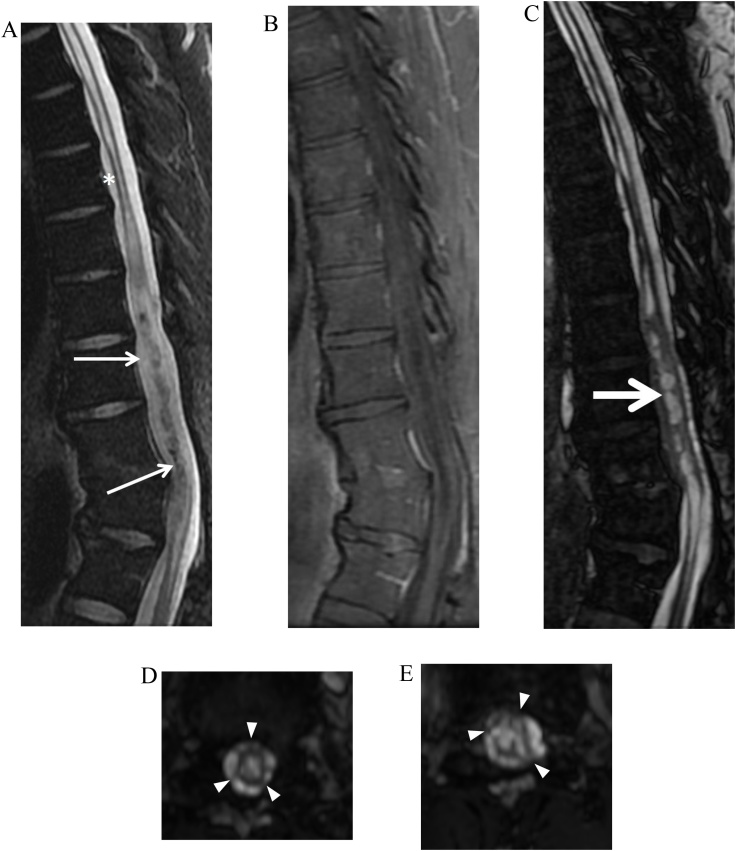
Arachnoid adhesions and complex syringohydromyelia. 34-year-old man with history of remote discitis osteomyelitis presents with worsening back pain, bilateral lower extremity weakness, and imbalance. Sagittal T2-weighted FSE MRI image (slice thickness, 3 mm) (a) demonstrates syringomyelia throughout the thoracic cord with myelomalacia cranially (asterisk) and relative expansion of the lower thoracic cord with central low signal (small arrows) which could be interpreted as a mass. Sagittal contrast enhanced T1-weighted FSE image (slice thickness, 3 mm) (b) demonstrates no abnormal enhancement. Sagittal (slice thickness, 1.2 mm) and axial FIESTA-C MRI images (c–e) clearly demonstrate the complex syrinx in the expanded lower cord with multiple internal septations (large arrow). Multifocal regions of arachnoid adhesion causing cord expansion are visualized (arrowheads).
